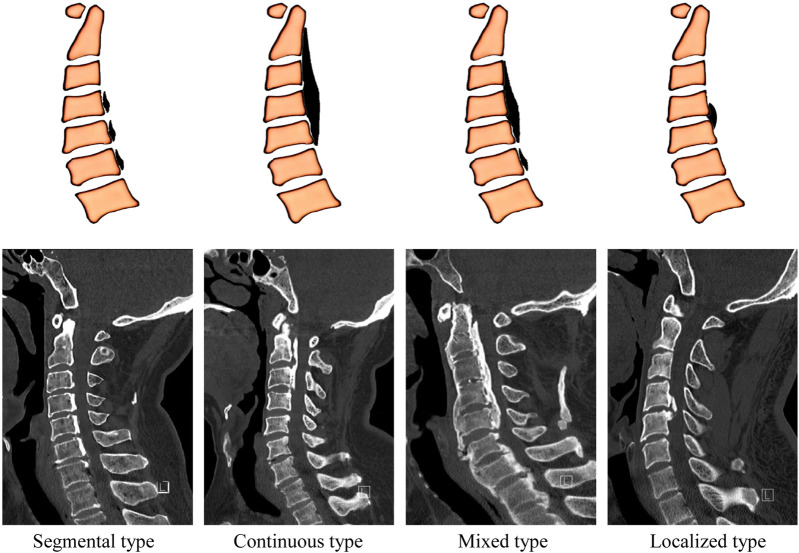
Imaging classification of OPLL. Sagittal CT scan images provided by Dr. Lai Jinquan, Shenzhen Luohu Hospital of Traditional Chinese Medicine.
